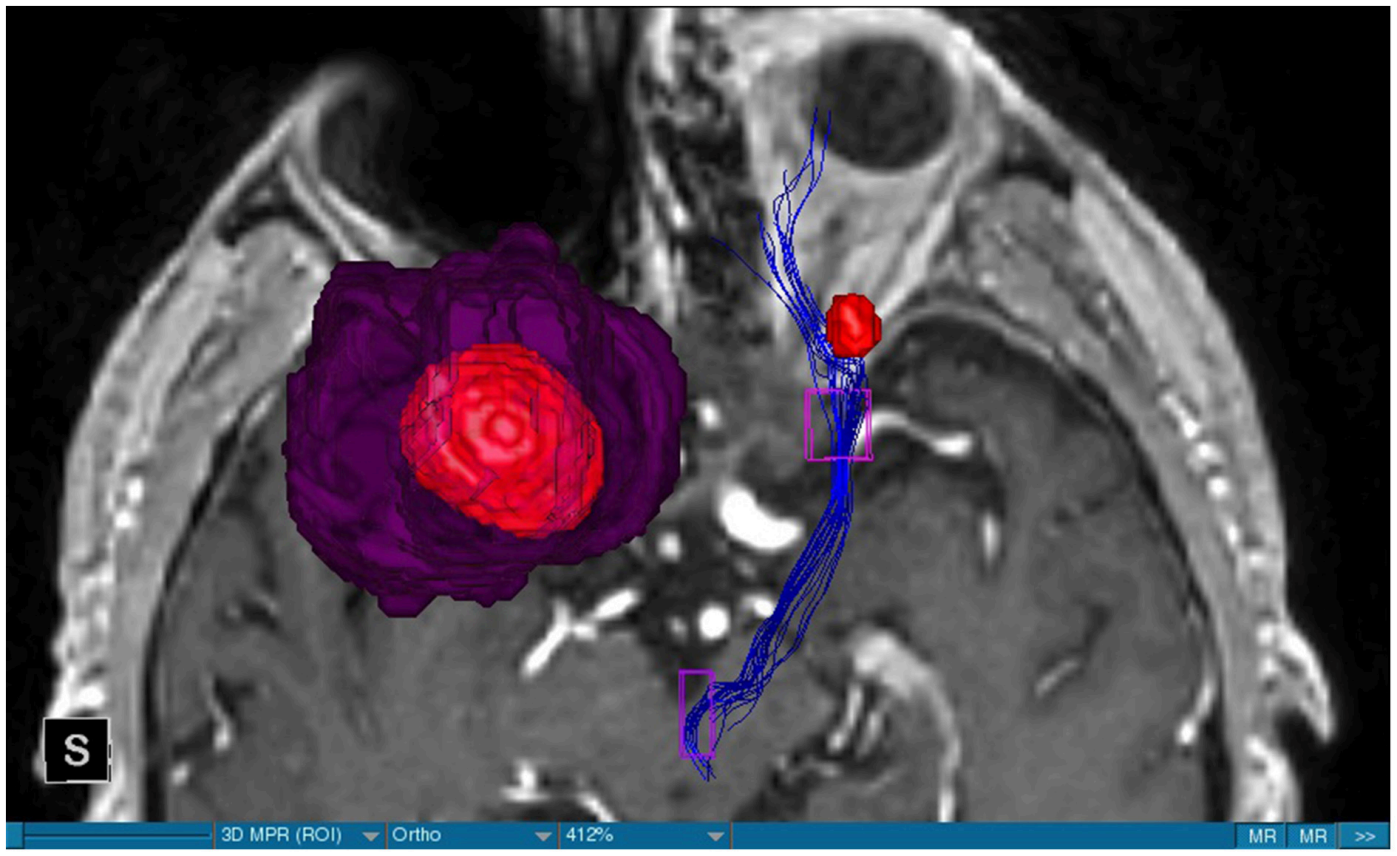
MRI axial view, T1, red: Metastasis, purple: edema, blue: right MFB, left MFB is missing.
